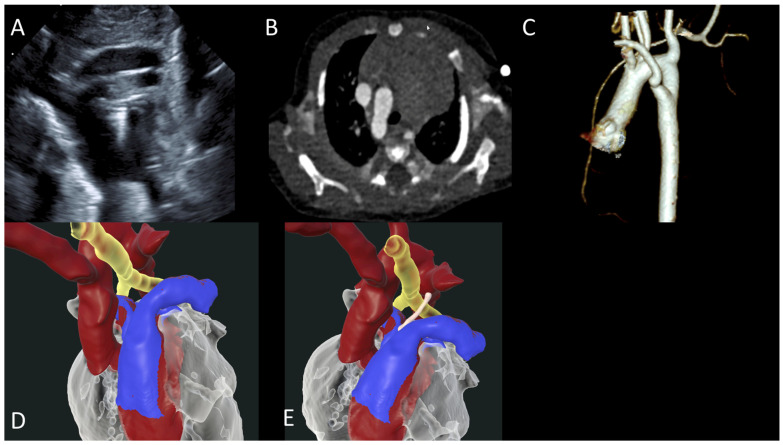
(A) TTE showing RAA; (B,C) CT indicating RAA with aberrant left subclavian artery compression of the trachea; (D,E) VR models demonstrating the spatial relationship between aorta (red), trachea (yellow), pulmonary artery (blue) and white represents ligamentum arteriosus completing the ring.
